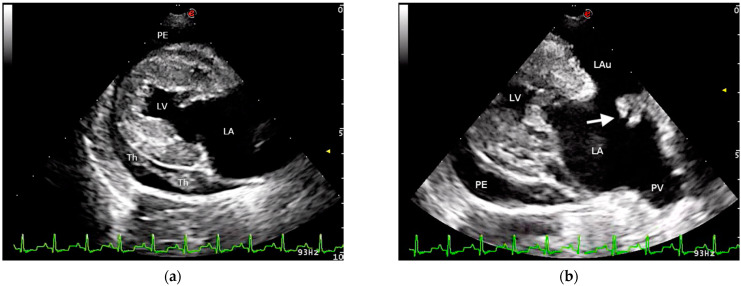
Conventional echocardiography at the presentation: (a) Right parasternal long-axis view showing pericardial effusion (PE) with hyperechoic density suggestive of a thrombus (Th). (b) Modified left parasternal long-axis view optimized to visualize the left atrium (LA) and auricle (LAu). A hyperechoic structure (arrow), adherent to the left atrial wall and consistent with a thrombus, is evident. LV, left ventricle; PV, pulmonary vein.
